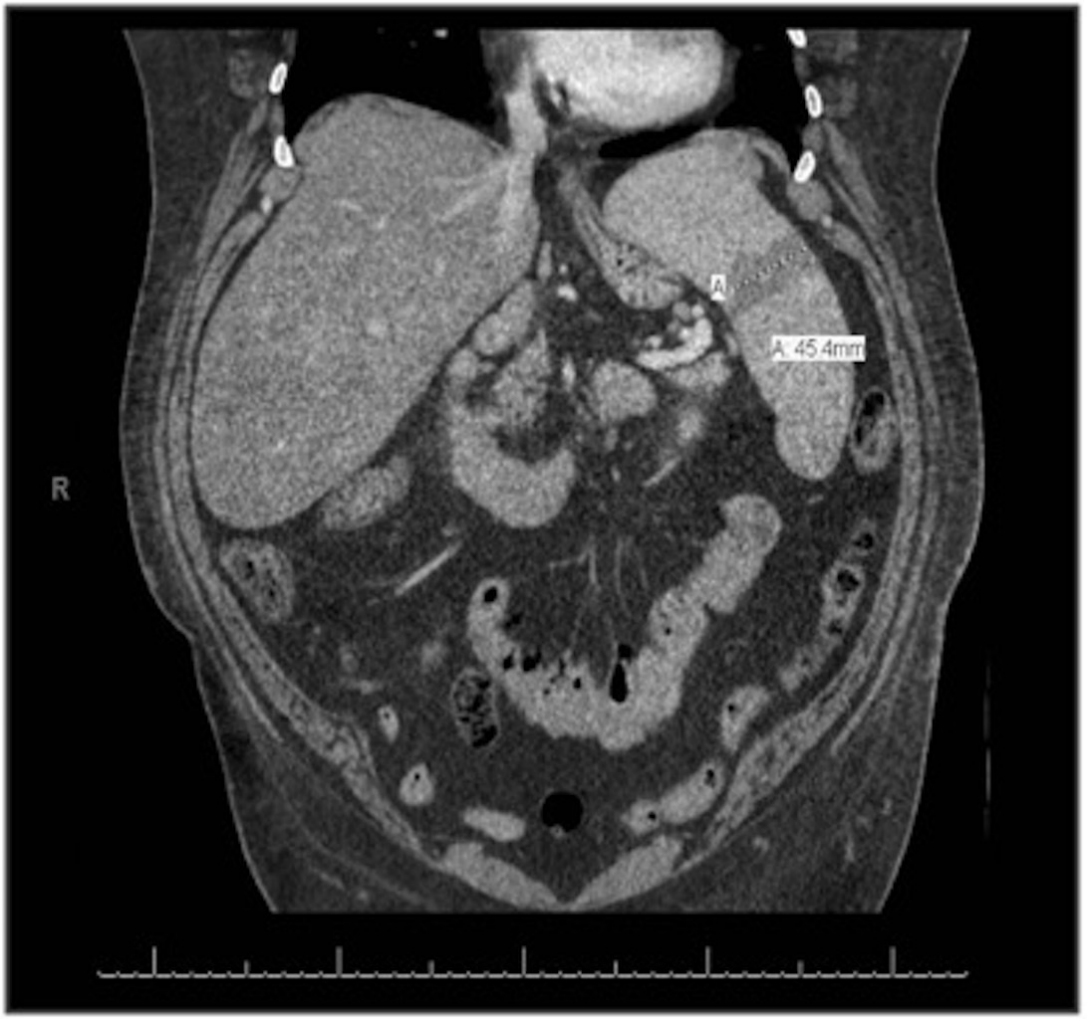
Acute splenic infarction on admission - CT-abdomen imaging. Hepatosplenomegaly and abdominal lymphadenopathy were also noted.
